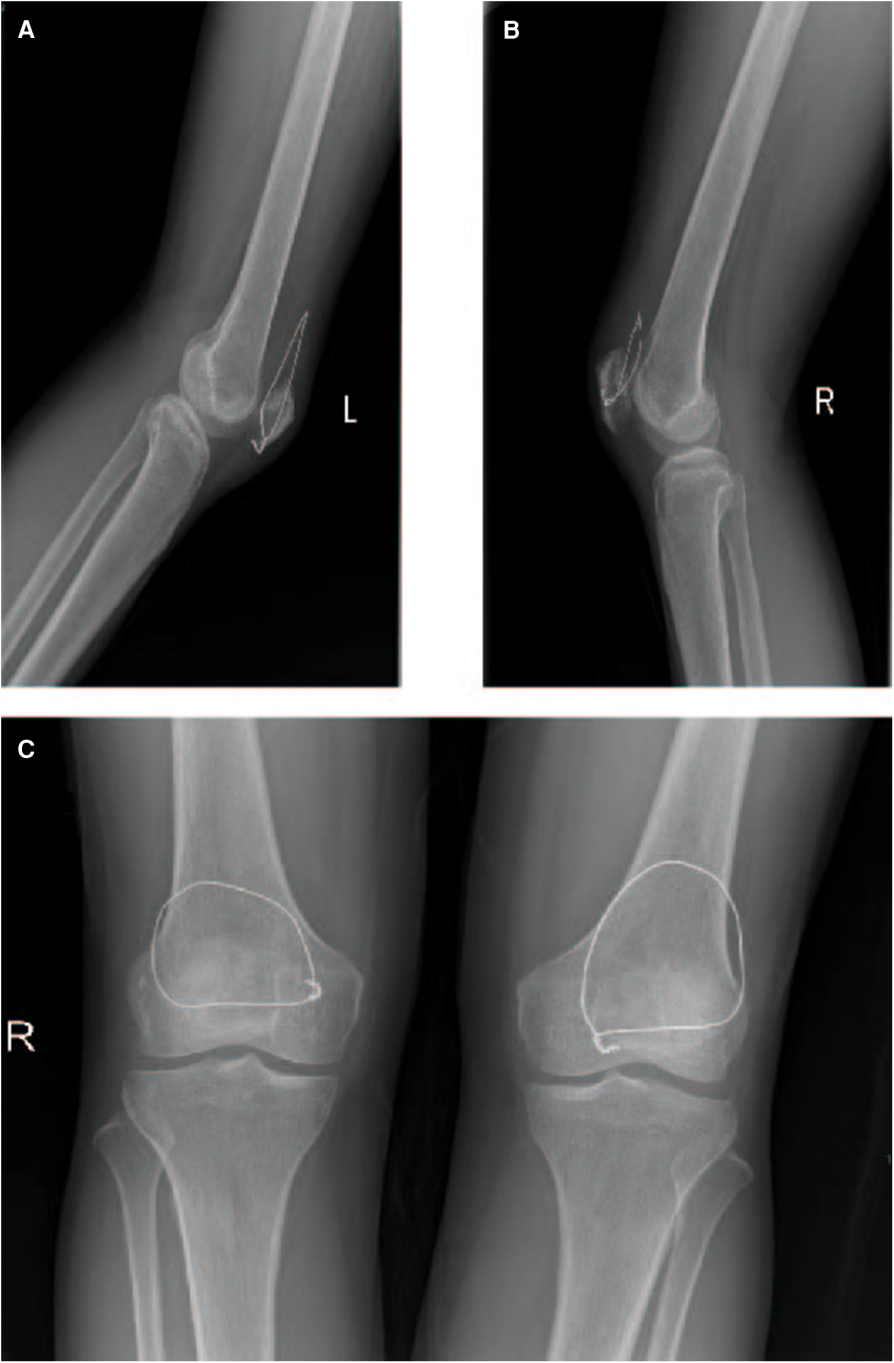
X-ray films of both knee joints were reexamined 3 months after operation. (A) Lateral x-ray of the left knee; (B) Lateral x-ray of the right knee; (C) Lateral x-ray of the left knee.
